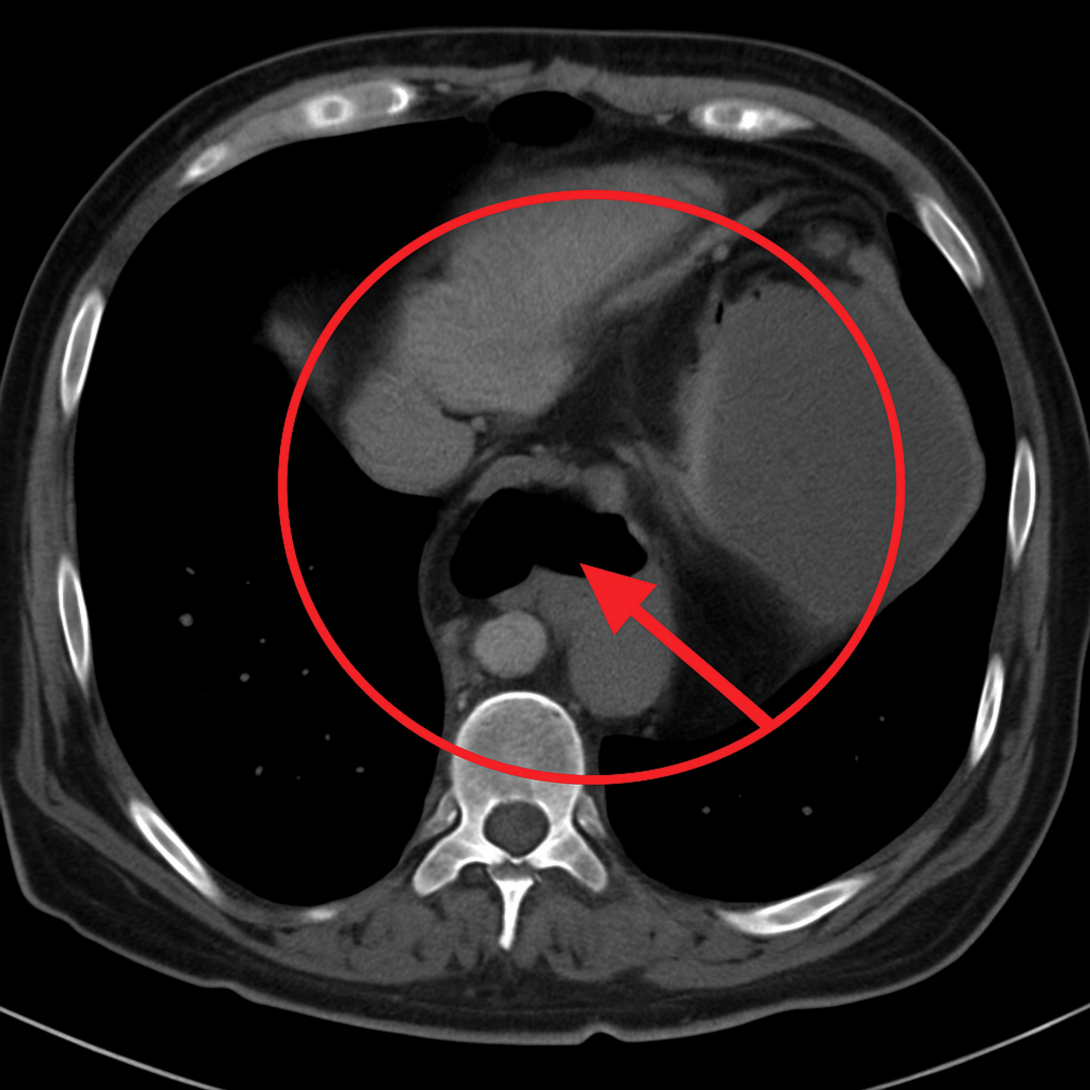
Axial contrast-enhanced computed tomography image at the level of the lower thorax demonstrates a diaphragmatic defect at the esophageal hiatus (red arrow), with intrathoracic herniation of the gastric fundus and body. The stomach appears dilated and rotated within the posterior mediastinum, consistent with organoaxial gastric volvulus associated with a giant hiatal hernia.
